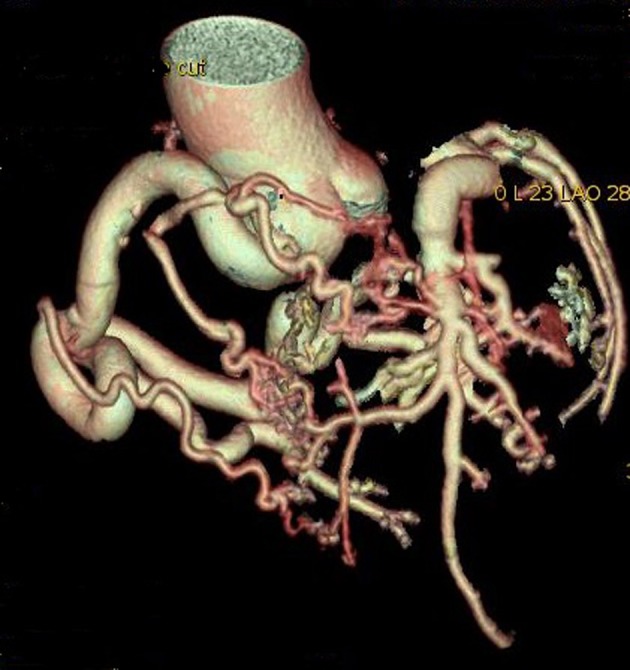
Reconstructed 3D CT angiogram showing dilated and tortuous right coronary artery with abundant intercoronary anastomoses communicating with left coronary artery and absence of its origin from aorta.
